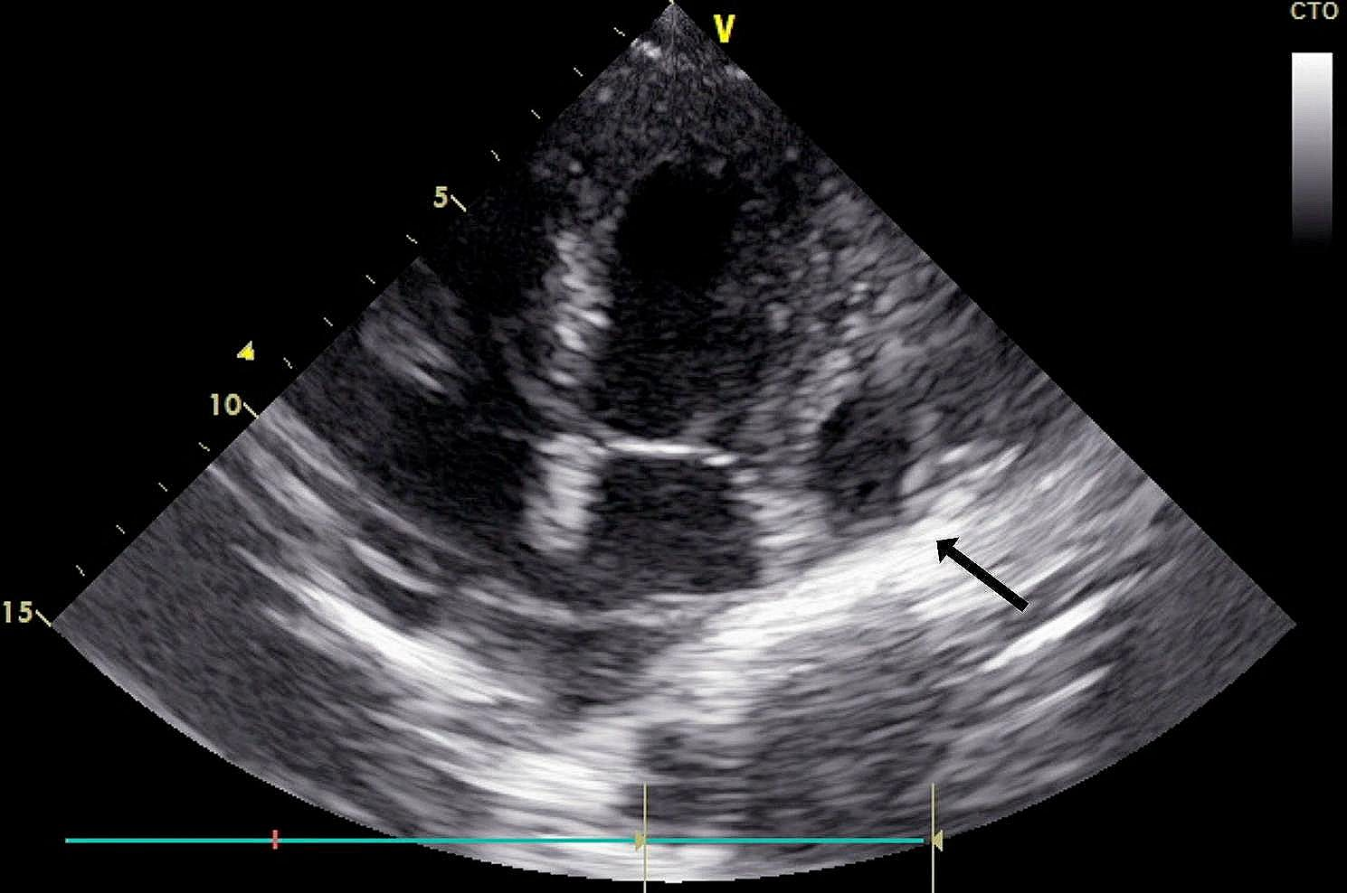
Echocardiographic apical 4 chamber view of the patient’s heart depicting the aneurysm lateral to the mitral valve
